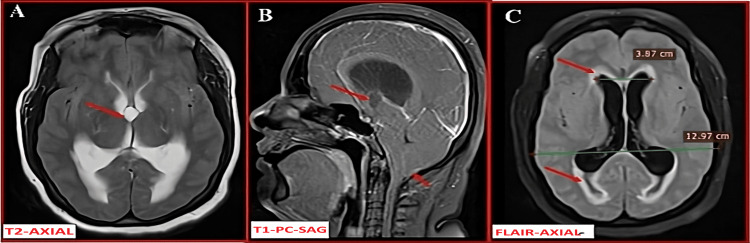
MRI brain images: (A) axial T2-weighted, (B) sagittal post-contrast T1-weighted, and (C) axial FLAIR images (A) Axial T2-weighted image shows a well-defined hyperintense cystic lesion at the roof of the third ventricle (red arrow), obstructing the foramen of Monro. (B) Sagittal post-contrast T1-weighted image demonstrates the non-enhancing nature of the lesion (red arrow) and inferior descent of the cerebellar tonsils below the foramen magnum, consistent with tonsillar herniation. (C) Axial FLAIR image reveals periventricular CSF ooze and dilated bilateral lateral ventricles (red arrows), suggestive of acute hydrocephalus (Evans index: 0.3). Note: All images are original and obtained from the institutional study dataset. FLAIR, fluid-attenuated inversion recovery
